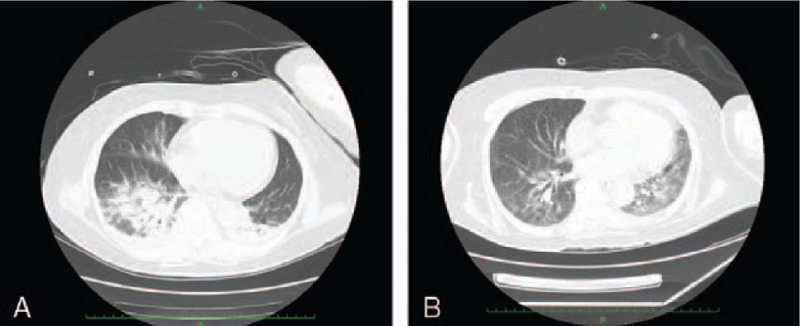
CT findings. (A) Lung CT on day 1. An infiltrative shadow due to pneumonia can be observed spreading to both lung fields. (B) Lung CT on day 84. An inflammatory ground glass opacity can be observed extending into the left lung field. CT = computed tomography.
